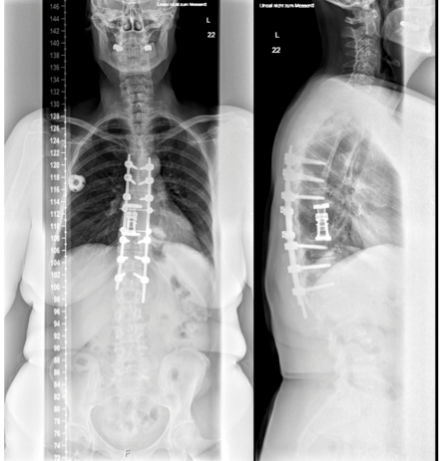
Postoperative X-ray (a.p. and lateral view). Posterior long segment pedicle screw stabilization and anterior reconstruction via expandable cage.
